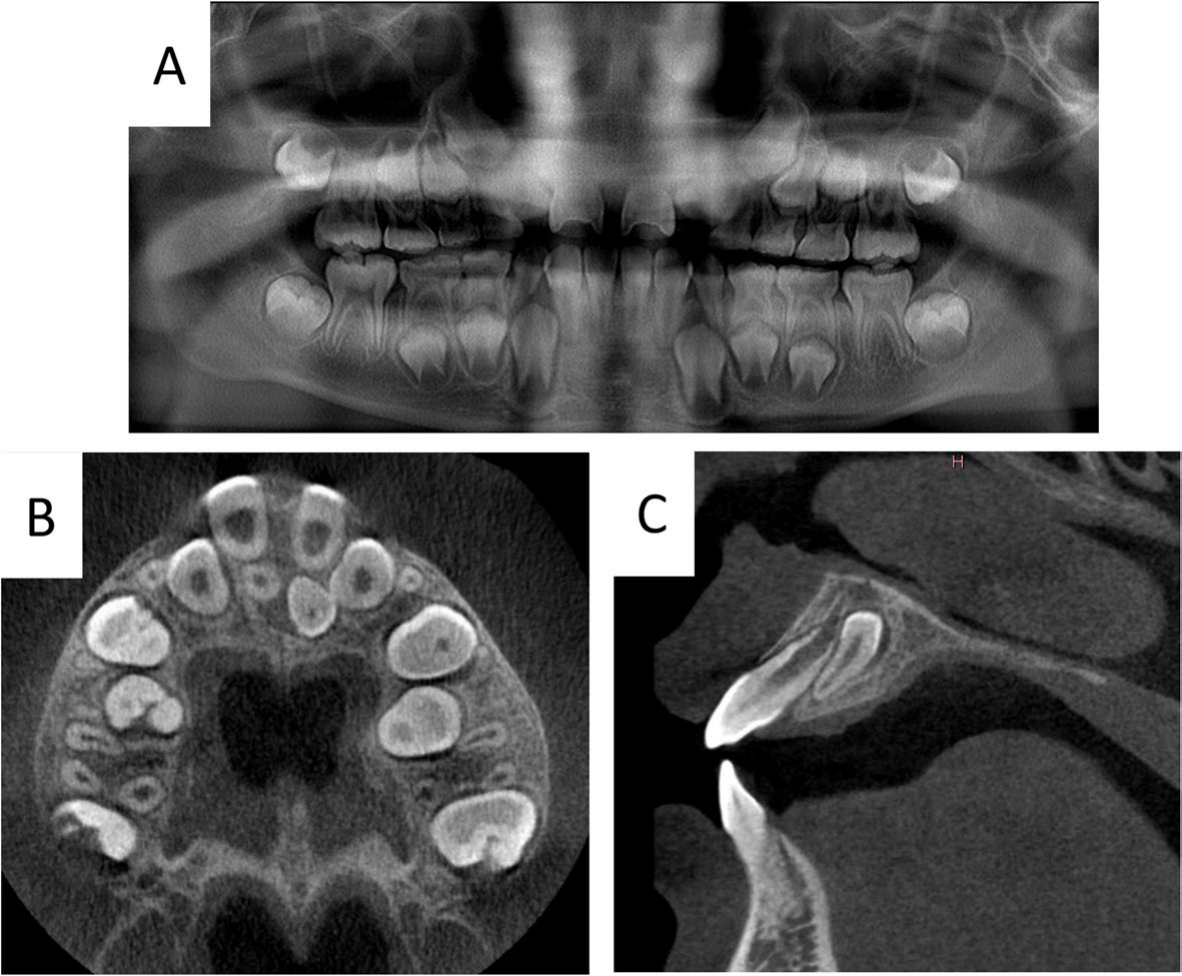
Case 1. A Panoramic radiograph. B CBCT axial view. C CBCT sagittal view
